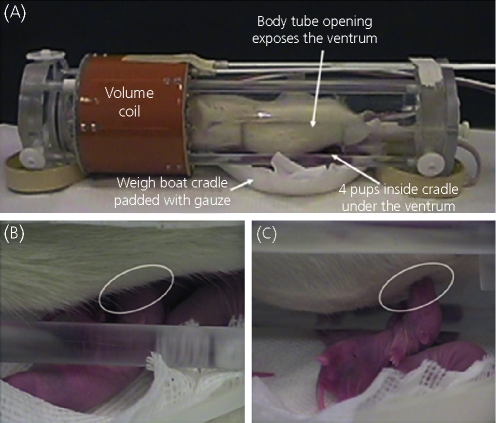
Photographs showing a conscious dam restrained in the device used for functional magnetic resonance imaging. A cradle of the dam's pups is positioned to allow access to the teats for suckling. © 2005 The Society for Neuroscience.
